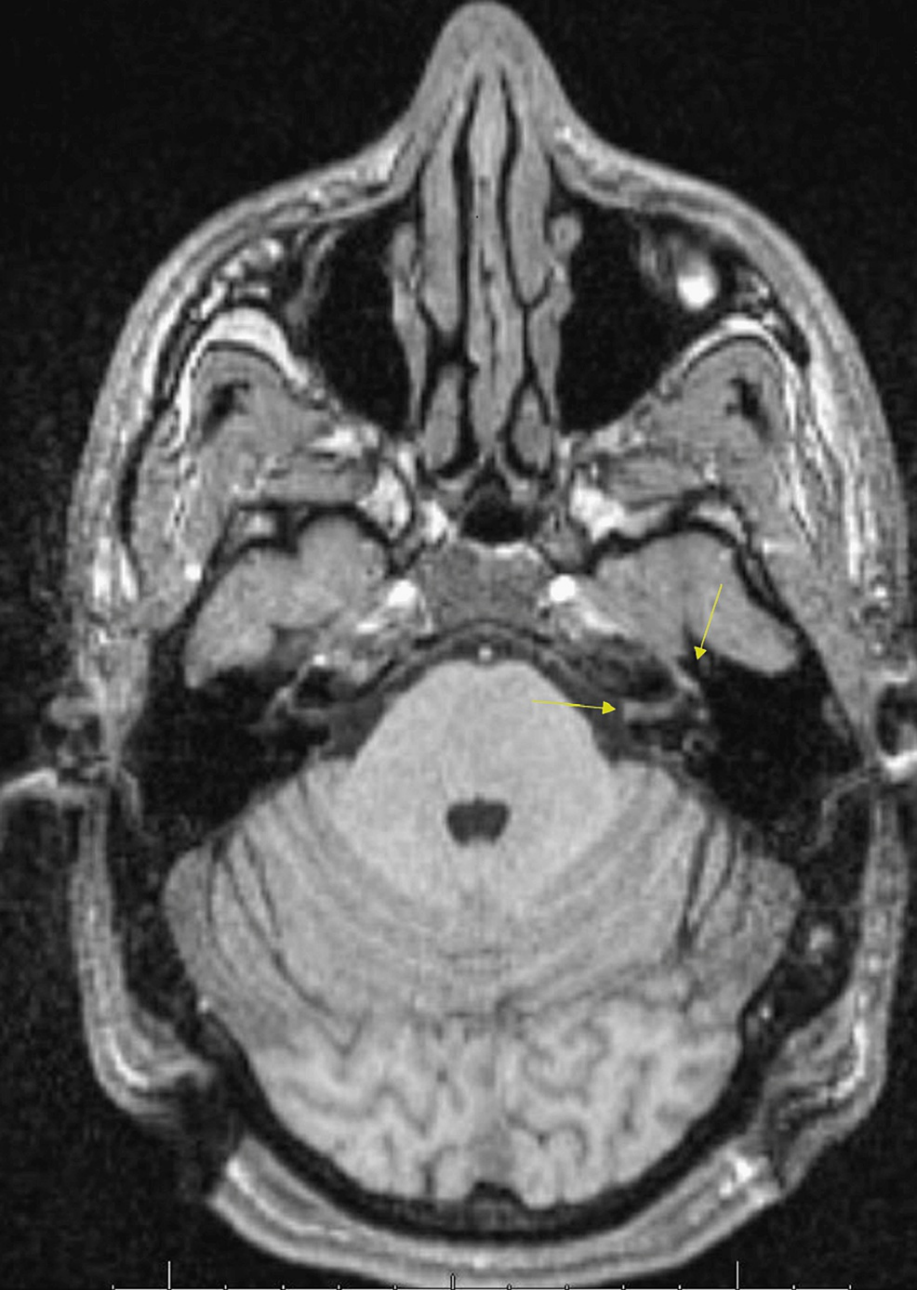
Axial T1 pre-contrast MP RAGE sequence MRI of the brain, pre-gamma knife procedure four months after initial imaging The image shows bright regions in the left porus acusticus as well as the left apex of the cochlea seen in the arrows above.
